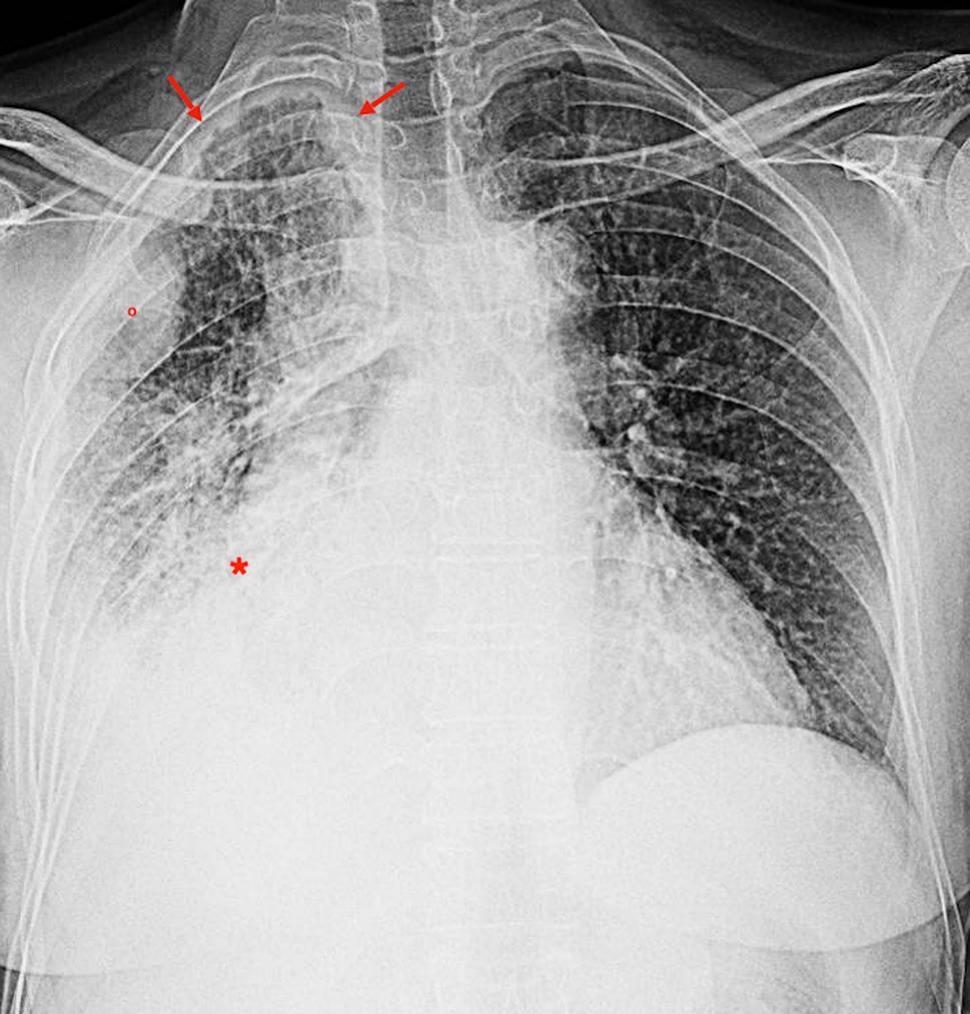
74-year-old man with history of occupational asbestos exposure and shortness of breath. Chest radiograph (frontal view) shows right-sided pleural effusion with consolidation of adjacent lung parenchyma (asterisk), pleural opacities (circle) and pleural thickening (arrows). Right lung volume loss and ipsilateral mediastinal enlargement can also be seen
